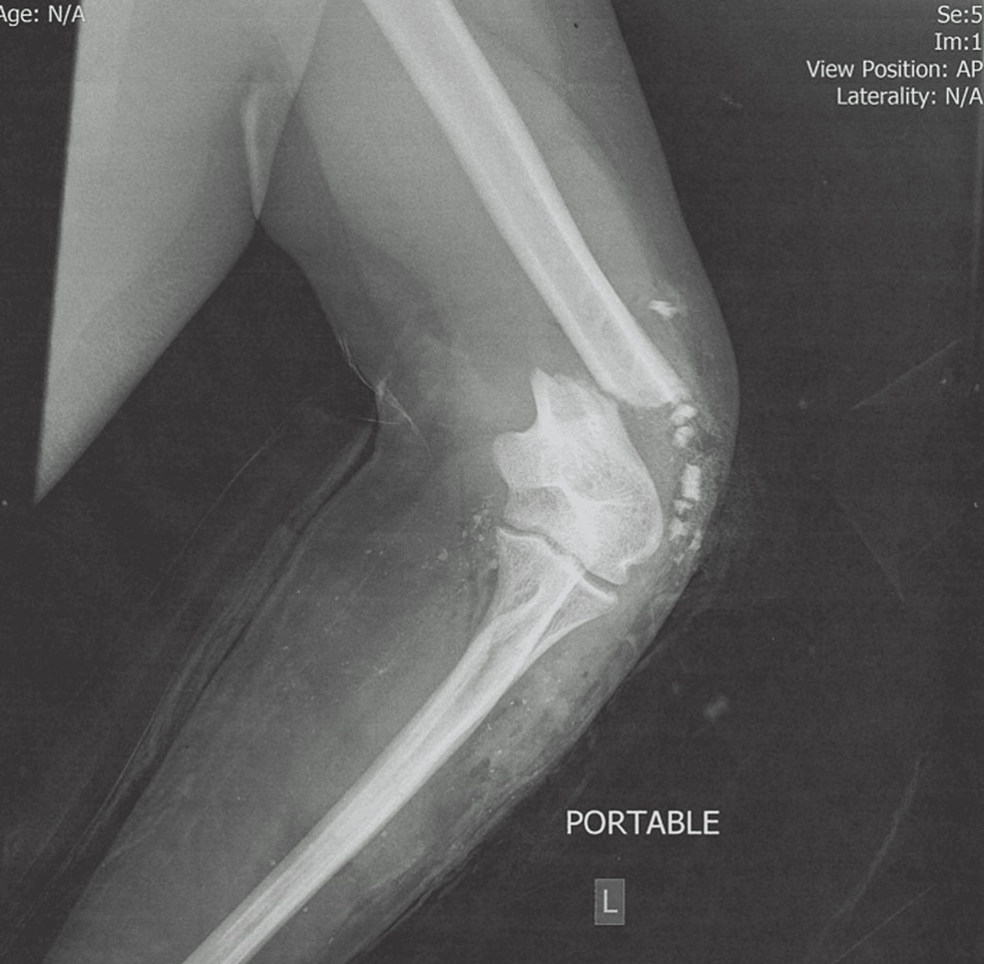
Initial X-ray after trauma demonstrating comminuted fracture of the distal humerus
